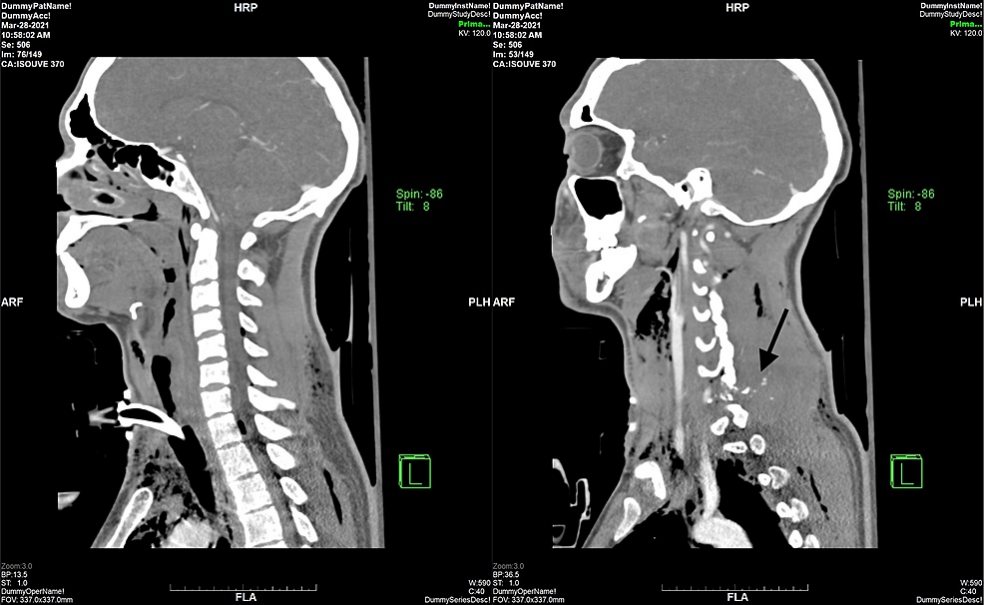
CT Images of the Cervical Spine (Sagittal view) Showing Tracheostomy Site and Multiple Comminuted Fracture Fragments (arrow)
